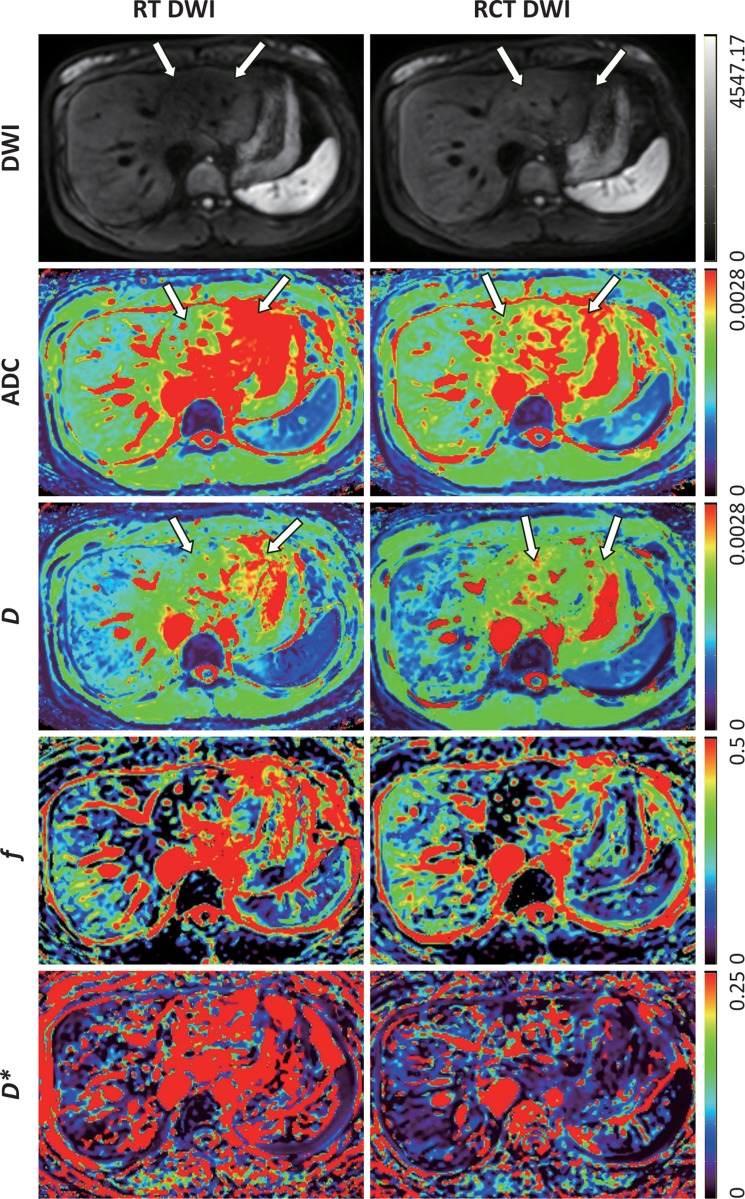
Axial diffusion-weighted MR images (*b* = 500 s/mm^2^) and ADC and IVIM parametric maps obtained by using RT and RCT scheme in a healthy 29-year-old woman The ADC and *D* values obtained with RT DWI were obviously higher in the left liver lobe (arrows) than those in the right. The differences of ADC and *D* values between the two lobes for RCT DWI were obviously lower than those for RT DWI. For RCT DWI, the *f* and *D maps of the liver showed relatively homogeneous, which indicated less variability of *f* and *D values compared with RT DWI.
